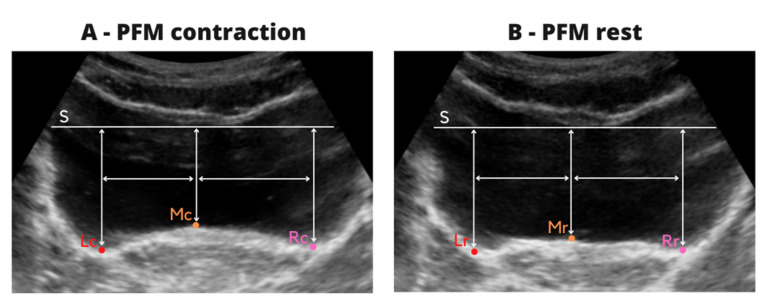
Description of ultrasound image analysis during contraction (A) and rest (B).
